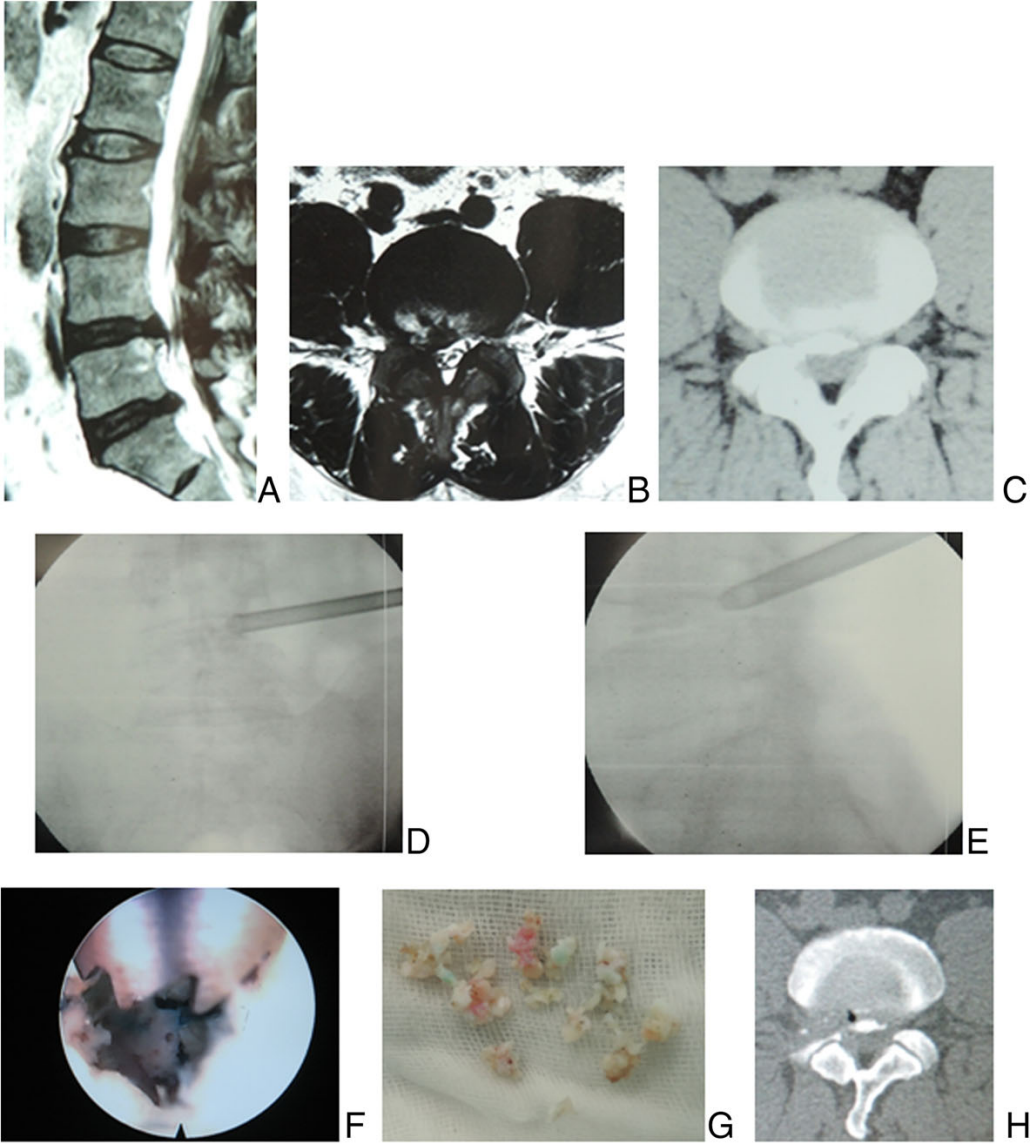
Male patient of 42 years with L4/5 disc herniation and calcification underwent the procedure of PTES. a Sagittal, b axial MR images, and c CT scan showed calcification in L4/5 disc herniation. During the procedure of PTES, d posteoanterior and e lateral C-arm image confirmed that a 7.5-mm working cannula was advanced directly to the protruding fragment. A 3.5-mm small reamer was used to grind off g calcified tissue under f view through the endoscope, which was confirmed on h CT scan after surgery
